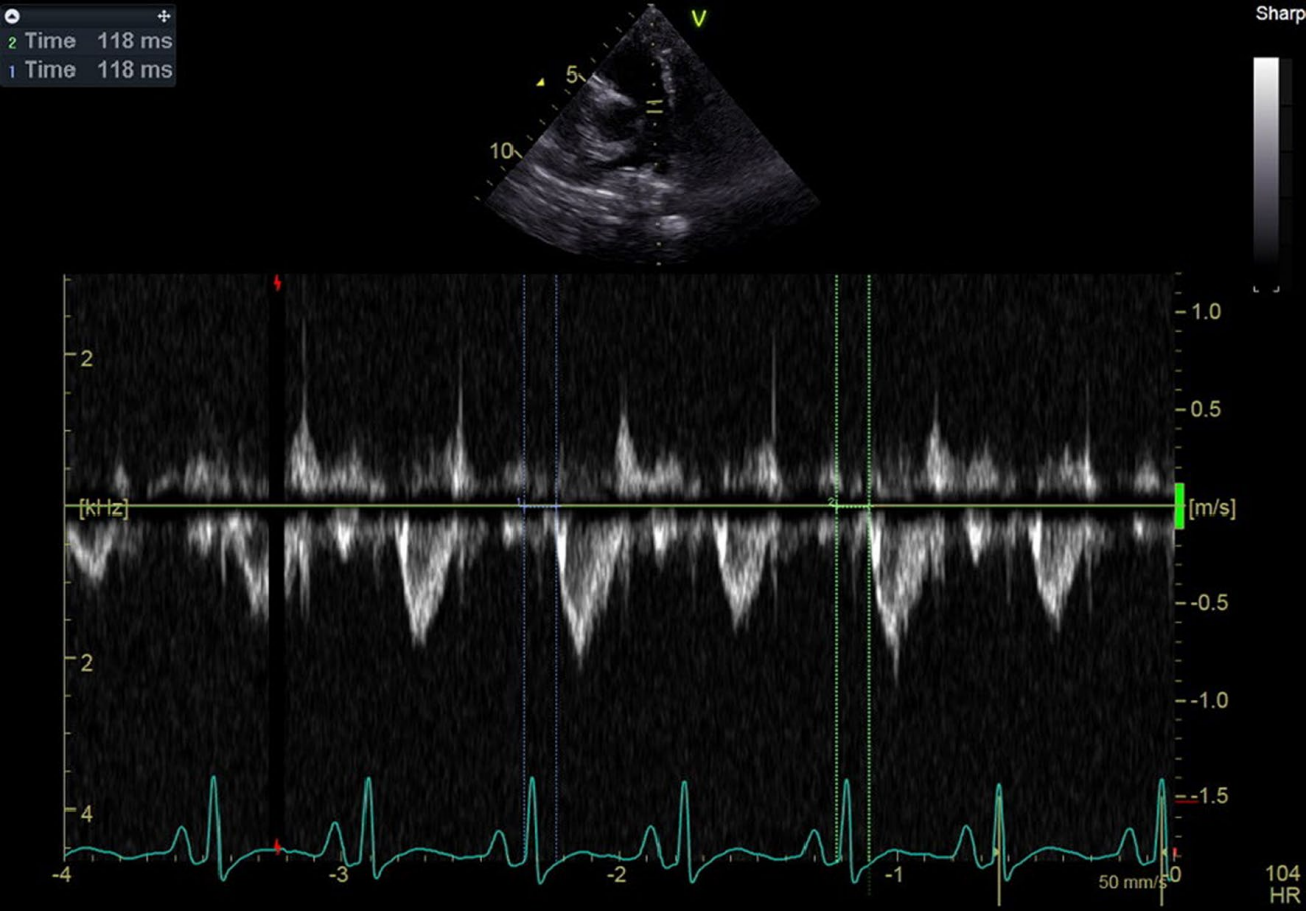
Electromechanical delay of RV by Doppler analysis = 118 ms
